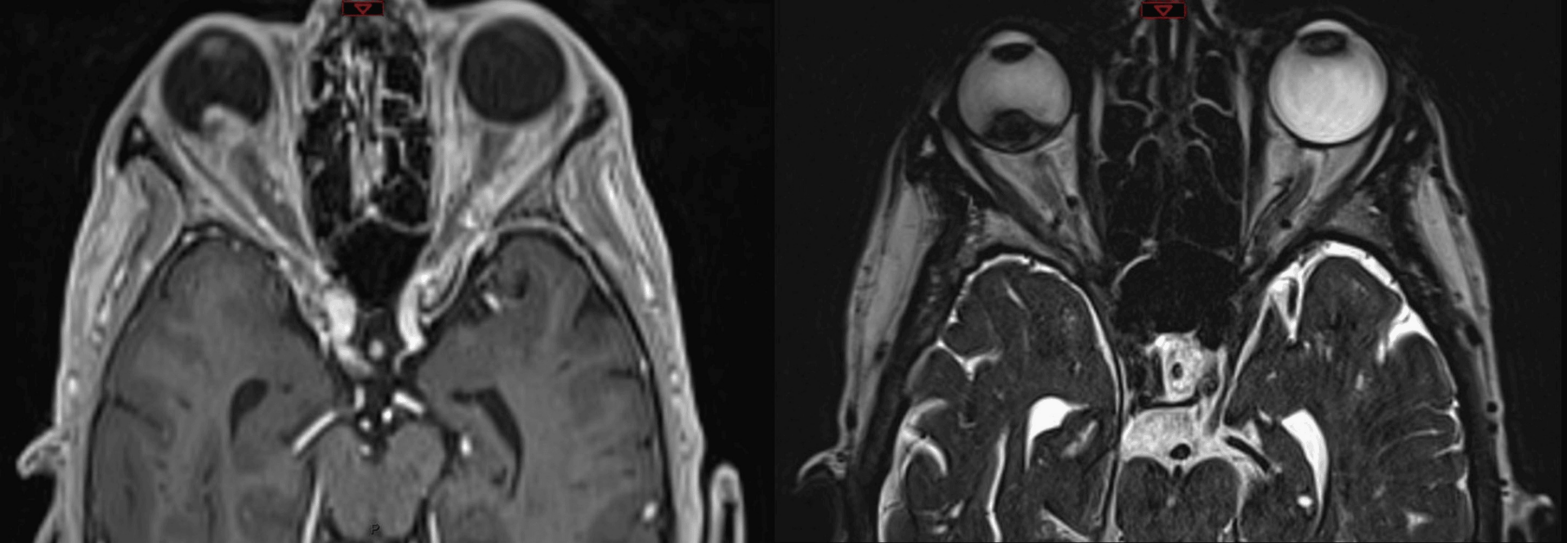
Magnetic Resonance Image - T1 (left), T2 (right). MRI images 6 months after treatment show a significant decrease in the size of the uveal melanoma in the right eye from the initial GTV of 1.47 cm^3^ to a GTV of 0.72 cm^3^, which is a reduction of 51%. GTV: gross tumor volume
